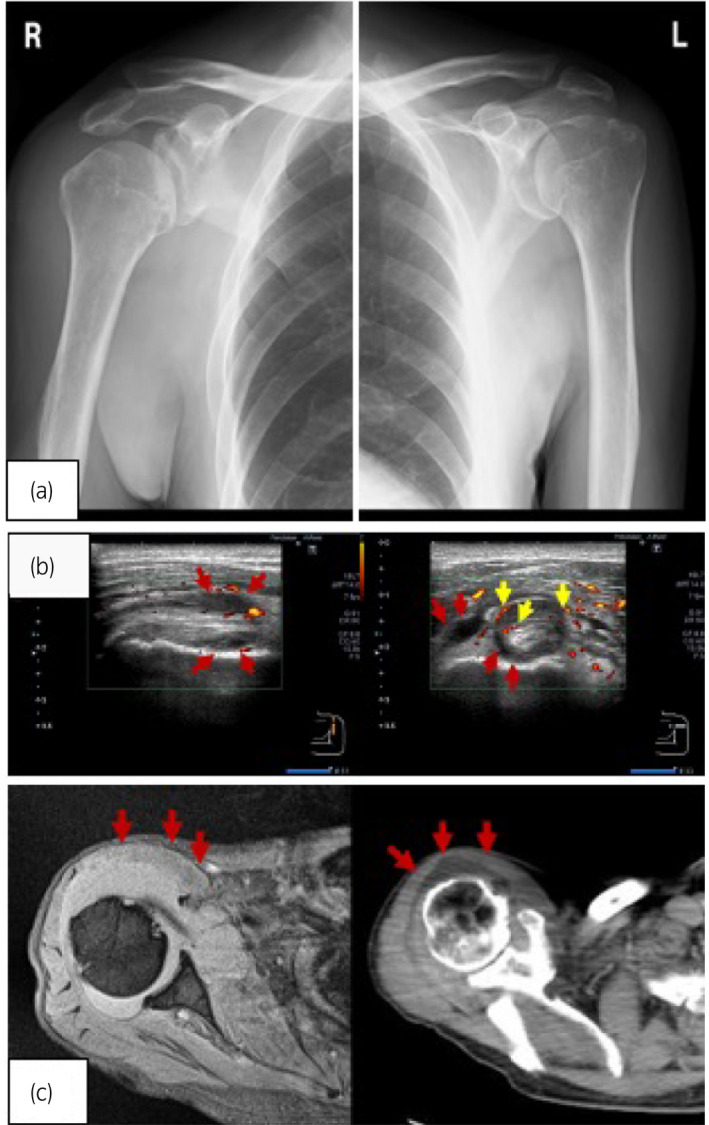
(a) Plain radiographs of the right and left shoulders showed no bone deformation, no marginal erosions, no localized osteopenia, or no fracture. (b) Doppler‐ultrasound revealed signs of tenosynovitis including synovial fluid (red arrows) of the biceps brachii and increased blood flow signal (yellow arrows). (c) Magnetic resonance imaging (left) and computed tomography (right) of the shoulder joint showed synovitis (arrows) without evidence of destructive images such as bone erosion.
